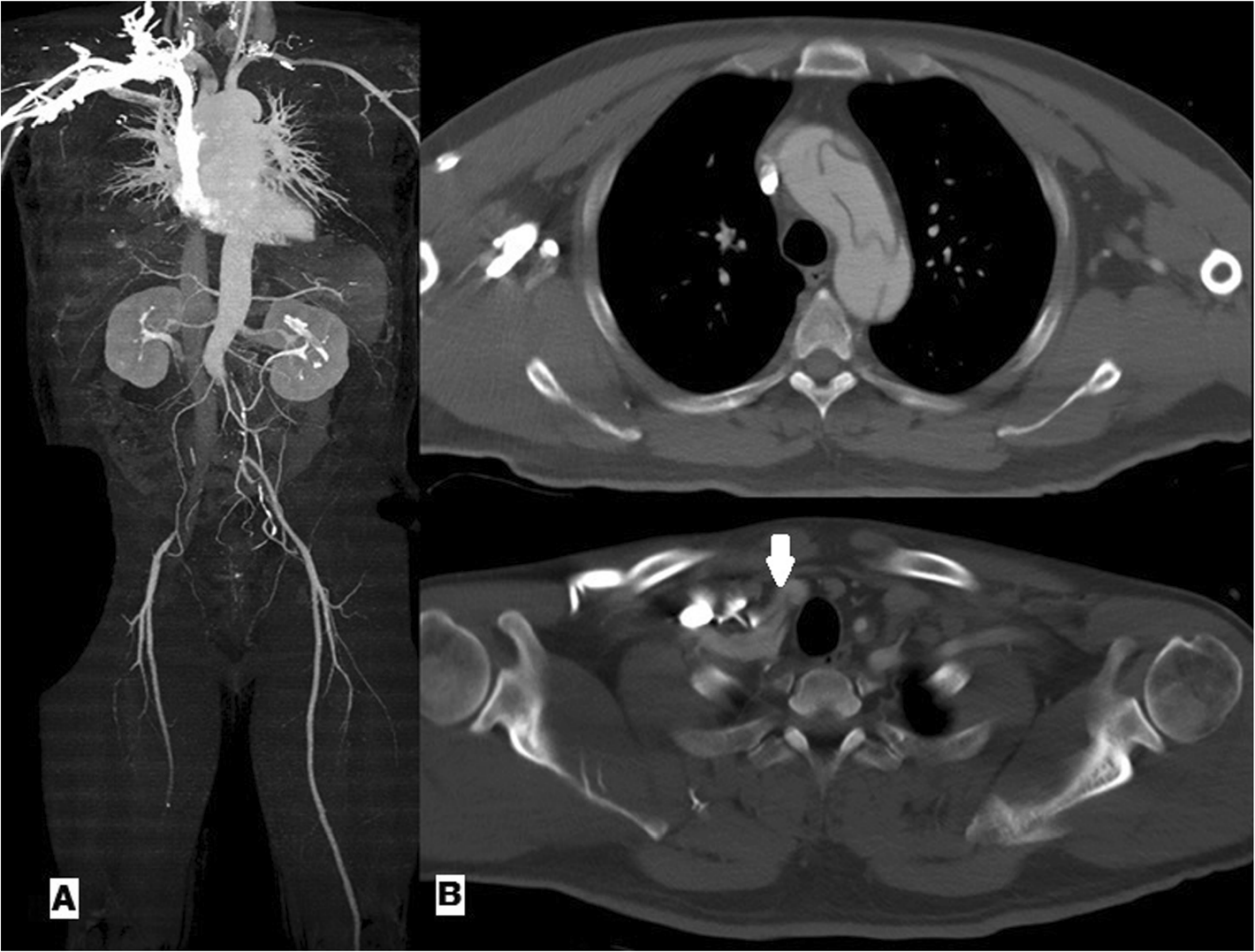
a: 3-Dimensional reconstructed aortic CTA showing occlusion of the infra-renal aorta. b: Acute type A aortic dissection involving the aortic arch and innominate artery (white arrow). CTA,computed tomographic angiography
